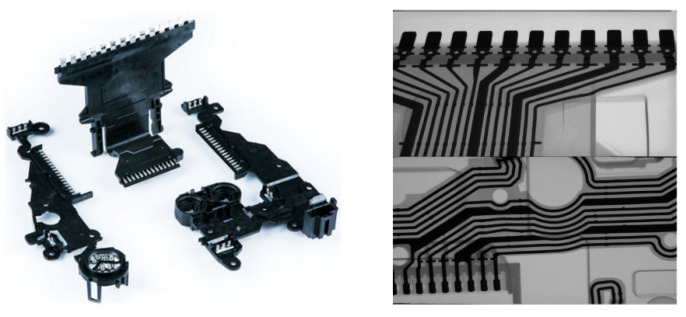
Lead frame over-molded (left) and its DR image under X-ray (right).
